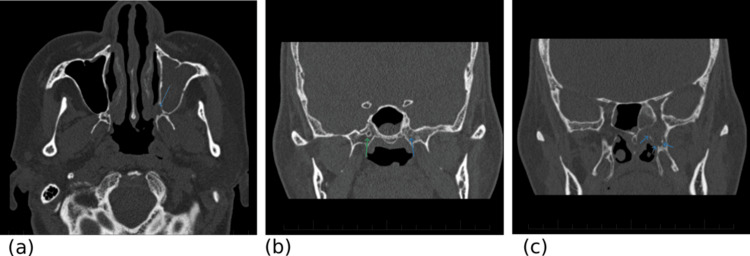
CT imaging demonstrating bony erosion consistent with perineural mucormycosis. (a) Axial CT showing widening of the left greater palatine canal (arrow), indicating early involvement of the greater palatine nerve. (b) Coronal CT demonstrating erosion of the anterior margin of the left vidian canal (blue arrow) with a normal right vidian canal (green arrow) for comparison. (c) Coronal CT showing erosion of the margins of the expanded left pterygopalatine fossa, the epicentre of the fungal perineural disease (arrows) CT: computed tomography
